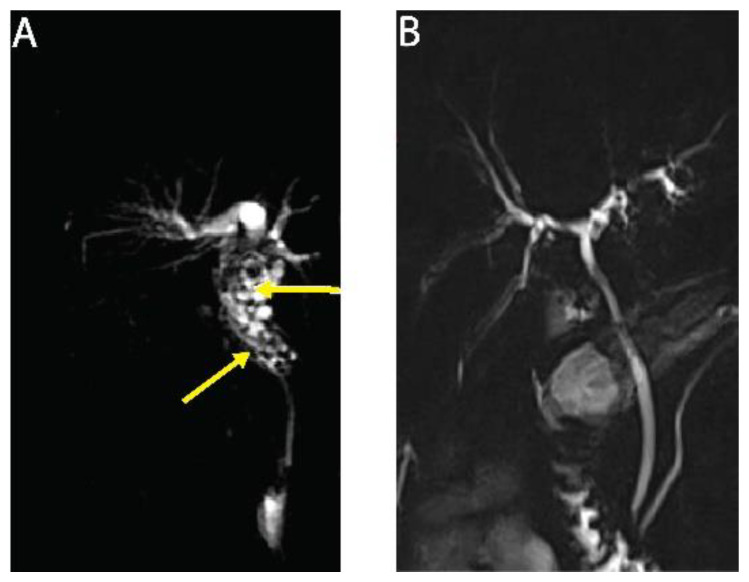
Cholangio-MRI images of human AE patient. (A) in July 2013, at time of diagnosis of a severe liver AE revealed by jaundice due to choledochus involvement. Numerous HyperT2 microcysts surrounding the upper part of choledochus (arrows). Above, note the enlargement of the intrahepatic right bile duct due to the impaired bile flow. (B) in February 2017 under ABZ therapy for 42 months. Complete disappearance of the hyper-T2 microcysts. In parallel, very favorable clinical and biological evolution, anti-Em18 antibodies negativation and perilesional activity disappearance on PET-CT evaluation (see Figure 1).
